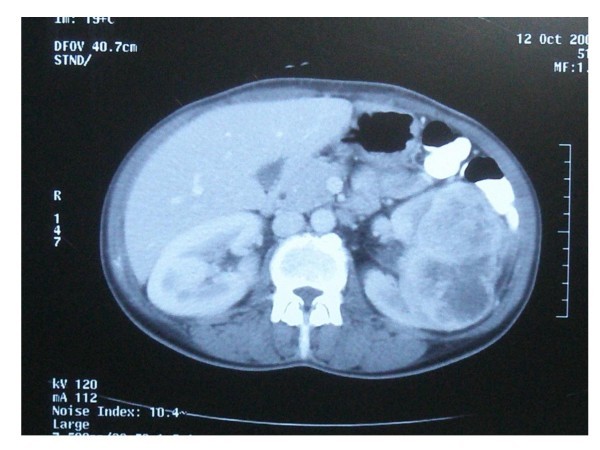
Presence of a left renal mass on abdominal computed tomography scan.
